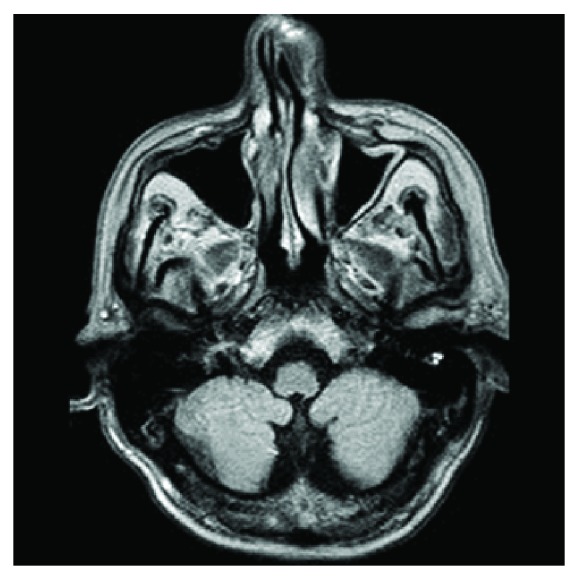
MRI scan 6 months after surgery.
